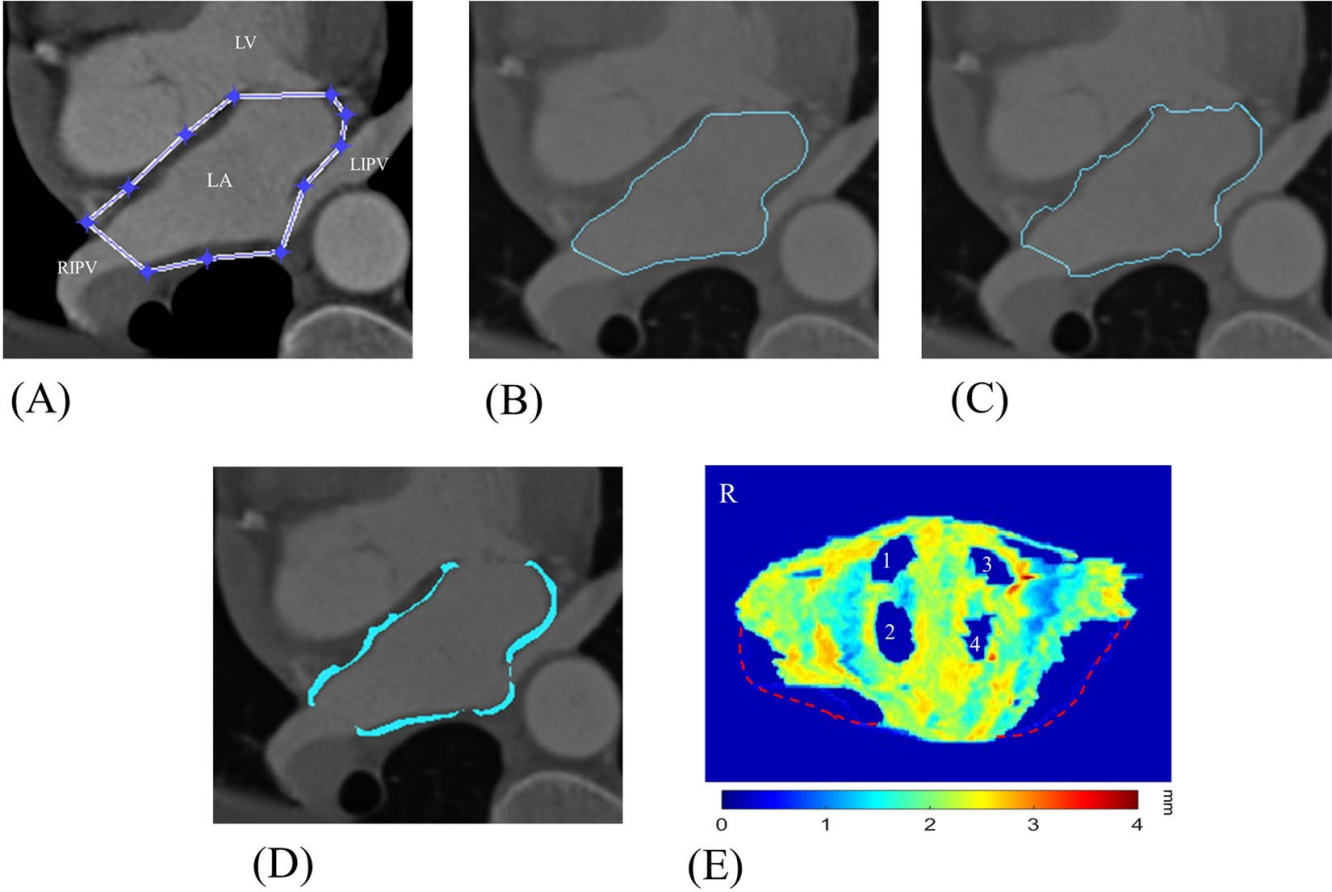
Procedures for processing and analysing a set of clinical CT images using the LA wall-mapping application. (A) LA delineation; (B) inner-boundary segmentation; (C) outer-boundary segmentation; (D) wall thickness calculation; and (E) 2D wall map generation. The colour bar shows the wall thickness. 1: right superior pulmonary vein (RSPV); 2 right inferior pulmonary vein (RIPV); 3 left superior pulmonary vein (LSPV); and 4: left inferior pulmonary vein (LIPV). The red dashed line indicates the annulus of mitral valve (MV).
